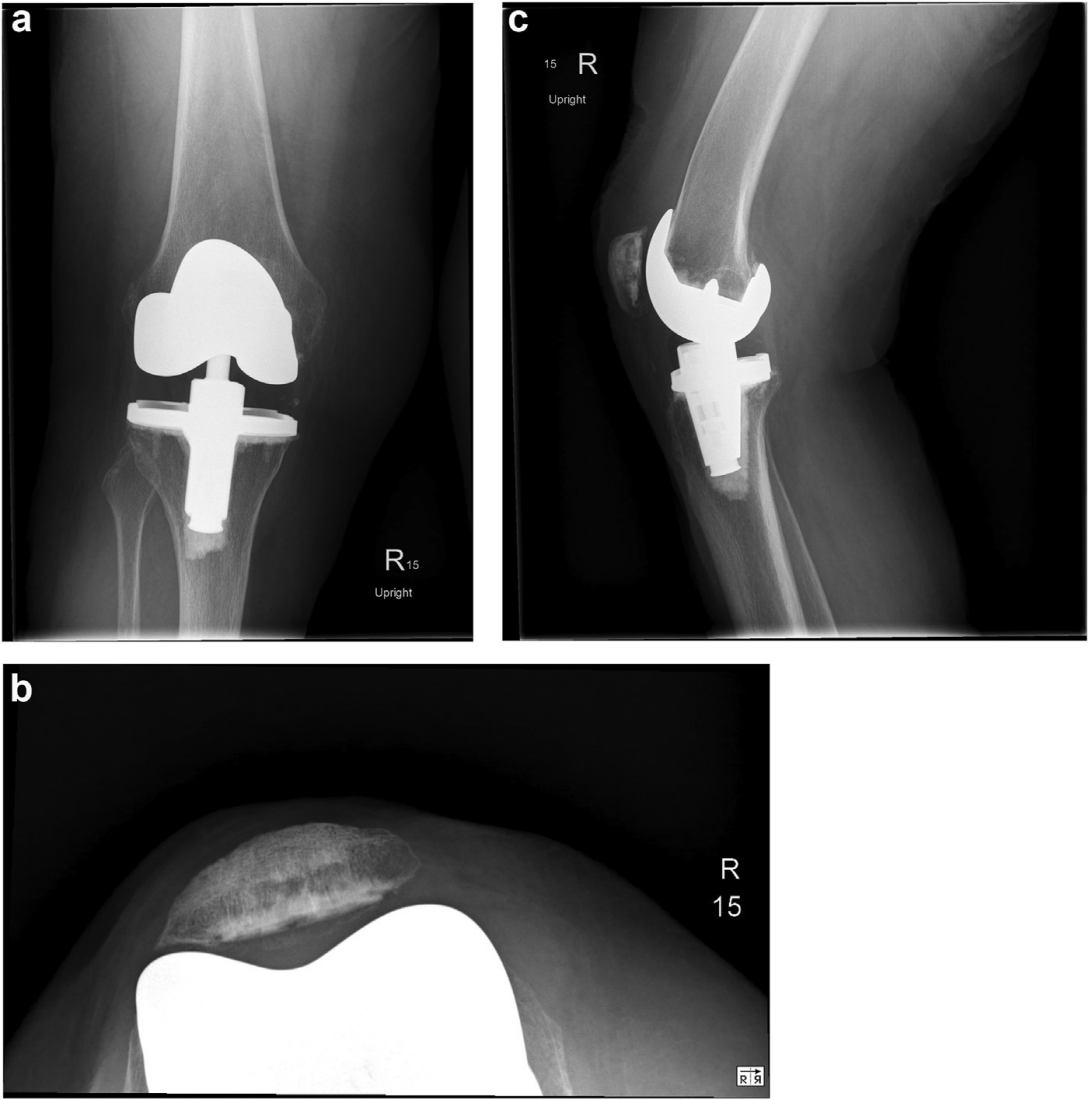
Second TKA revision at 2-year follow-up. (a) AP, (b) Merchant, and (c) lateral radiographic views demonstrate satisfactory alignment.
